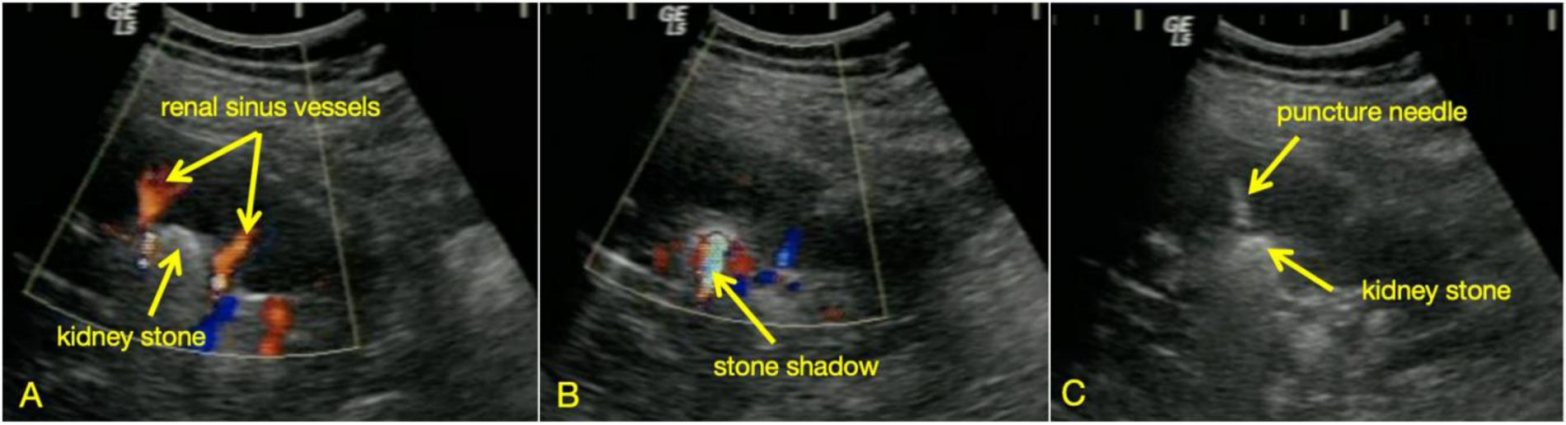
(A) Dopplar-mode shows preserved perfusion in the renal columns (normal vasculature), but hypovascularity at the fornix. (B) A prominent posterior acoustic shadow is noted deep to the calculus, suggesting possible adjacent prominent vasculature (originating from the renal columns) near the stone. (C) Switch off Doppler to optimize needle visibility during puncture.
